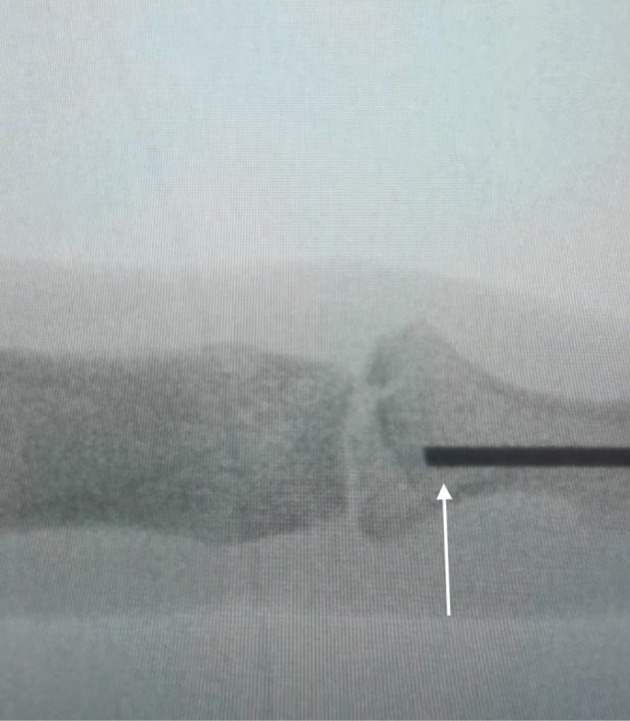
Intraoperative radiograph showing insertion of blunt end (white arrow) of K‐wire into the distal phalanx and applying it like a bone tamp.
